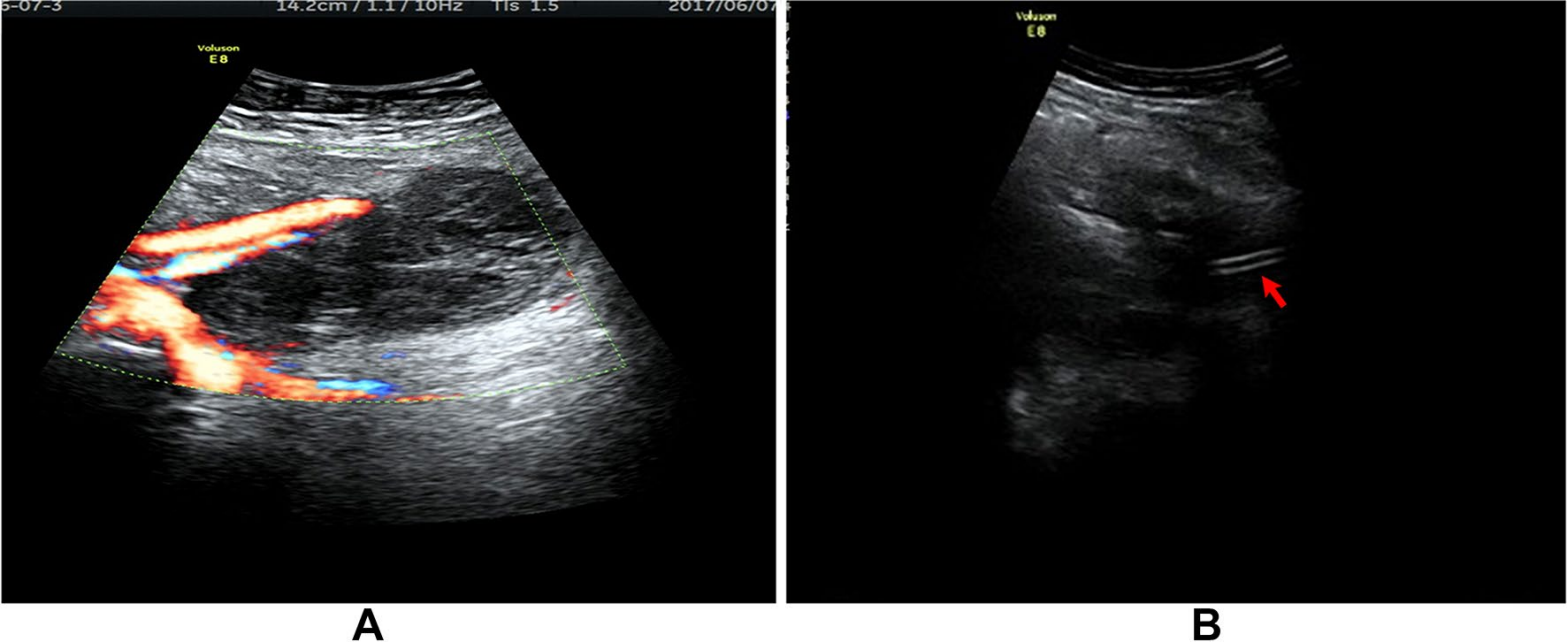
Ultrasonography showed thick-walled cyst with turbid content in mixed acho along the pelvic wall surrounded by iliac blood vessels (a); diminished lymphocele after percutaneous catheter drainage (red arrow: catheter) (b)
